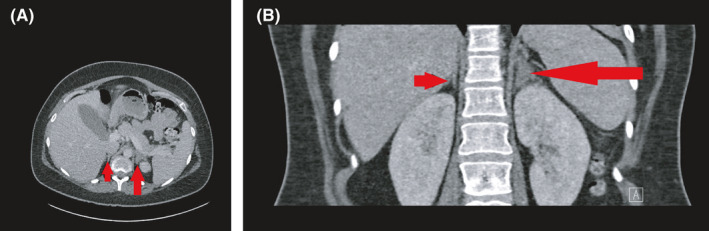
(A) Contrast‐enhanced CT adrenal gland in the axial view. The arrow clearly shows an enlarged and edematous left adrenal gland compared with the right. (B) Contrast‐enhanced CT adrenal gland in the coronal view. An enlarged and edematous left adrenal gland can clearly be visualized compared with the right
